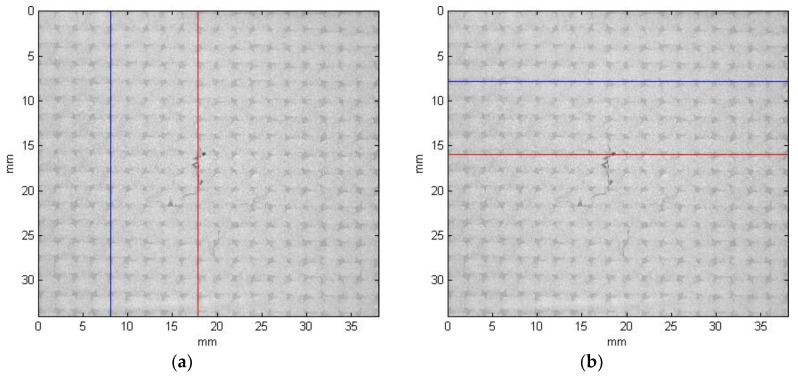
Positions of exemplary data vectors (not passing and passing through damage) in CT slices: (a) columns no. 368 and 814; (b) rows no. 357 and 726.
